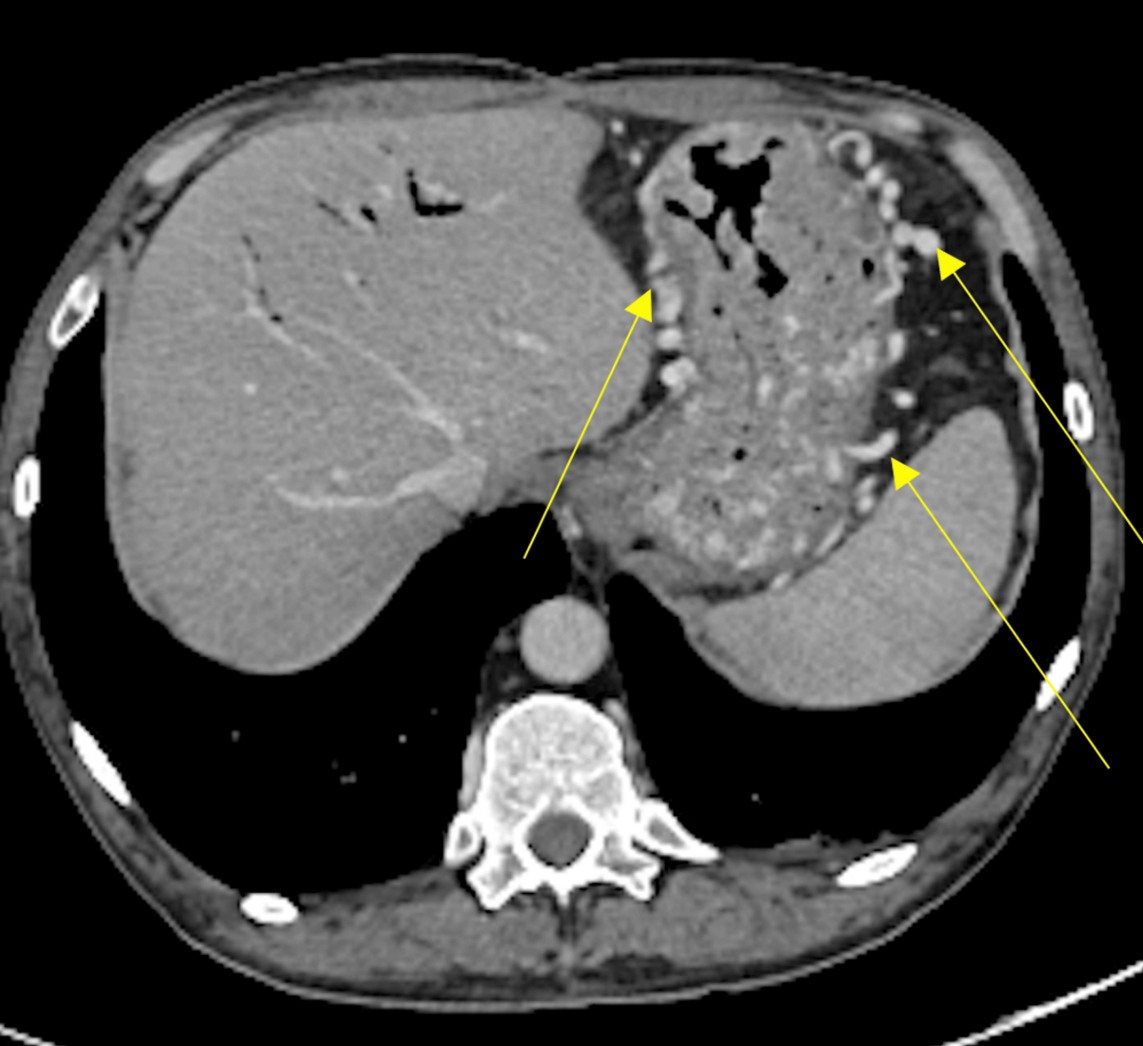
The axial view of the CT scan reveals IGV1 and IGV2 before splenic artery embolization. IGV1: isolated gastric varices type 1; IGV2: isolated gastric varices type 2
